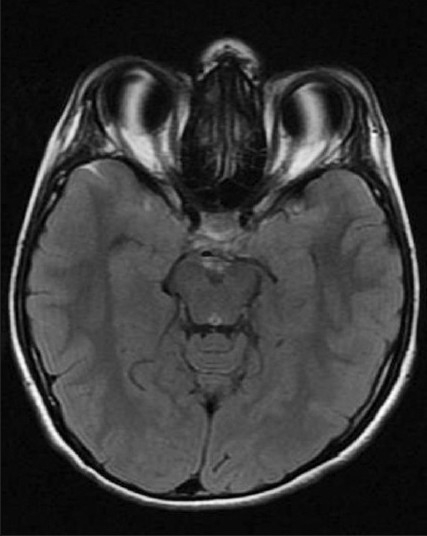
T1-weighted MRI axial view showing a pituitary tumor completely filling the sella in a child
